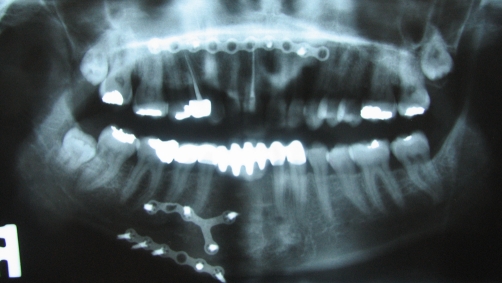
Panoramic radiograph after the restorations cemented.
